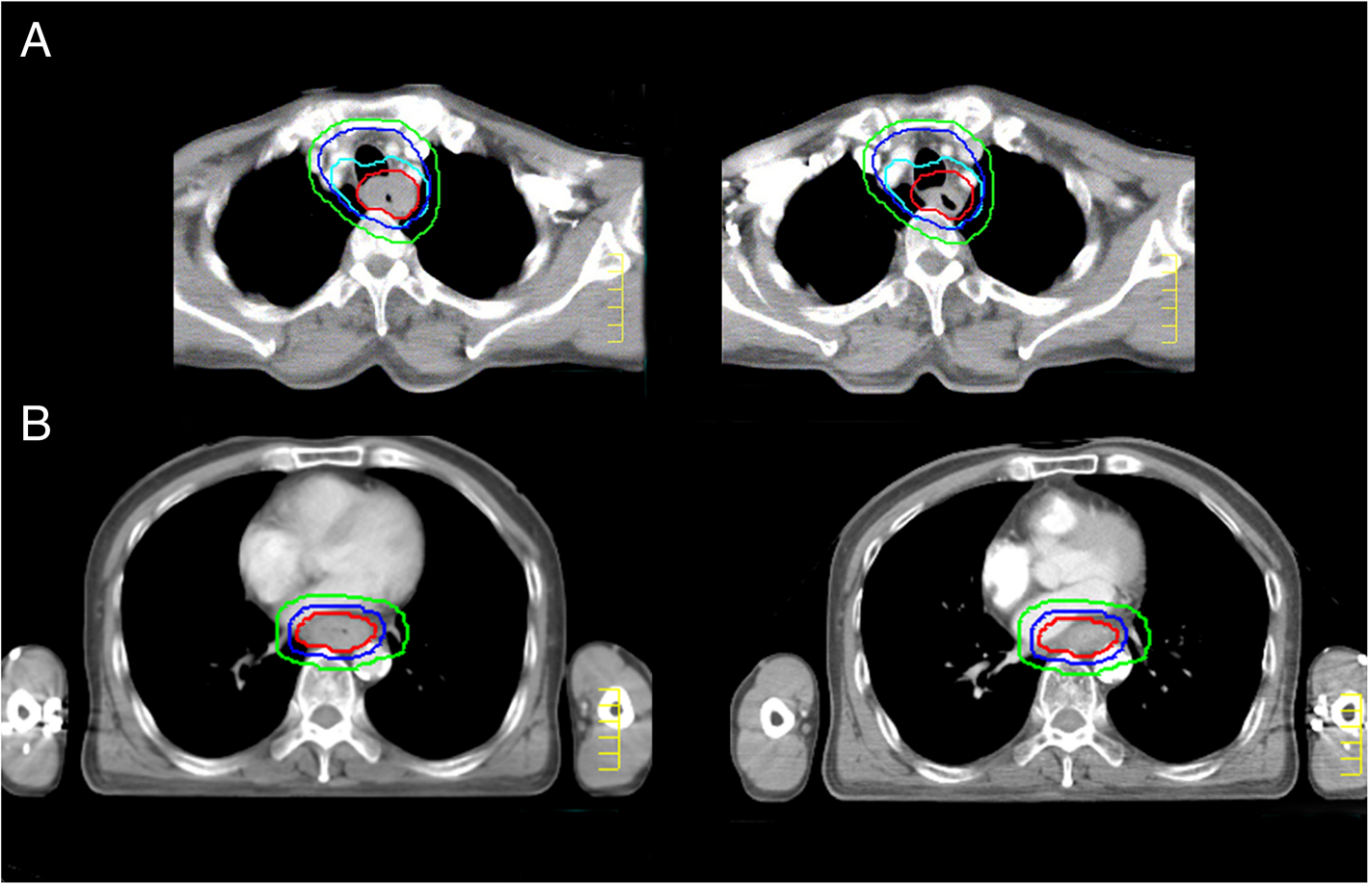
CT-CT fusion images of two enrolled patients. Images on the left are pre-treatment ones, while those on the right are taken after 23 fractions of radiation. Red line indicates GTV-T, the green line outlines PTV, the blue line outlines PGTV
